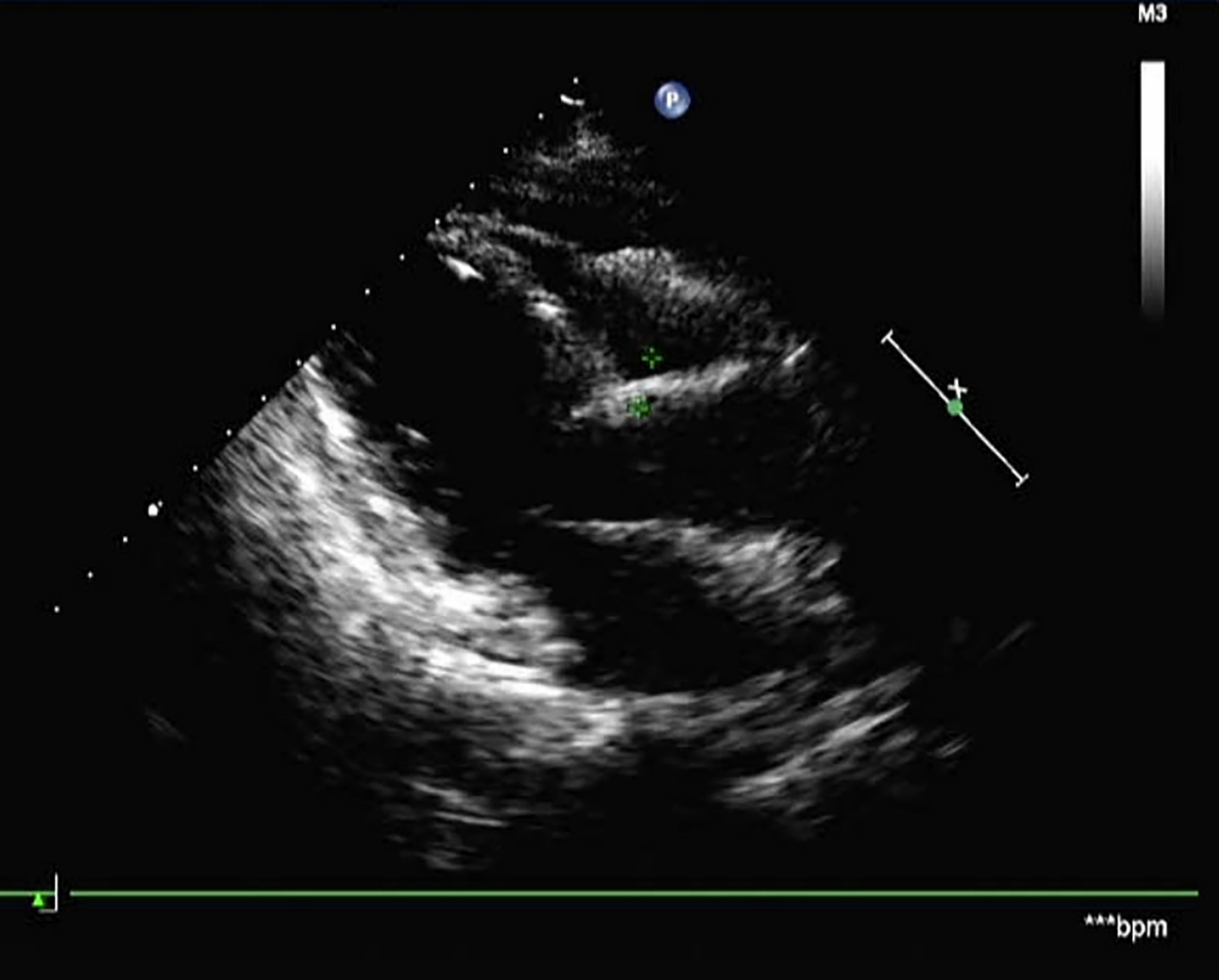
Postoperative echocardiography showed no residual tumor tissue and a normal cardiac chamber structure.
